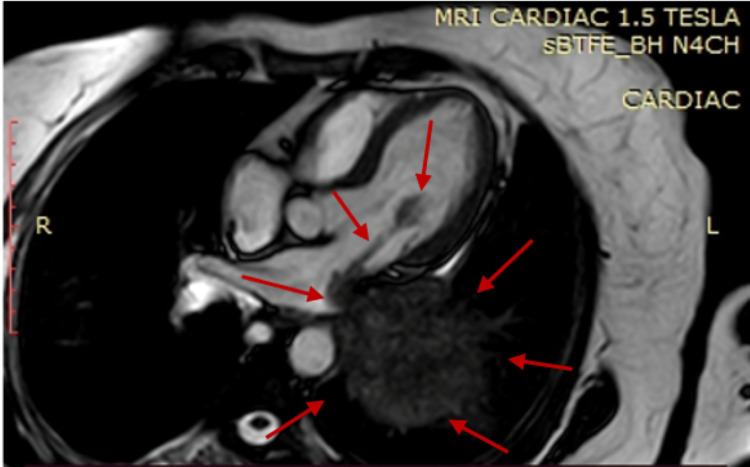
Cardiac MRI showing the left lung lower lobe mass (11 cm x 11 cm x 8 cm) entering the left atrium through the pulmonary vein, elongated left atrial mass (5.6 cm x 1 cm) inside the left atrium
